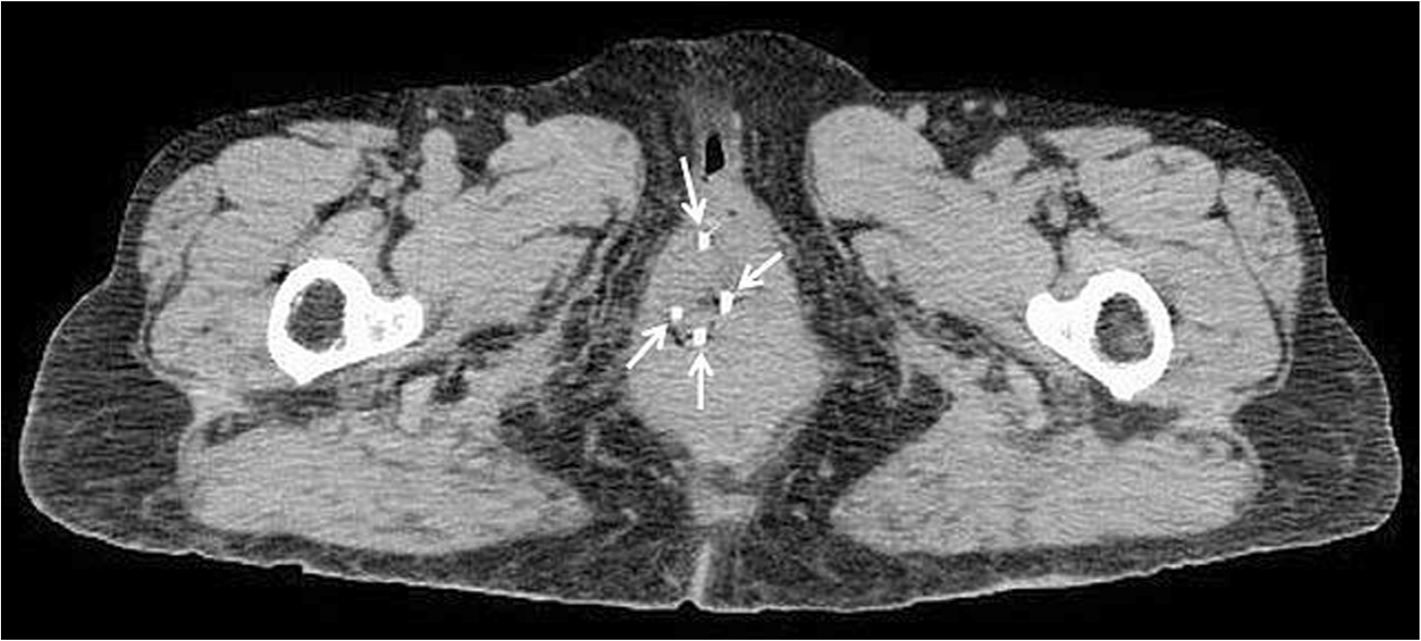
Axial computed tomography scan demonstrating four interstitially implanted dummy sources (white arrows)
